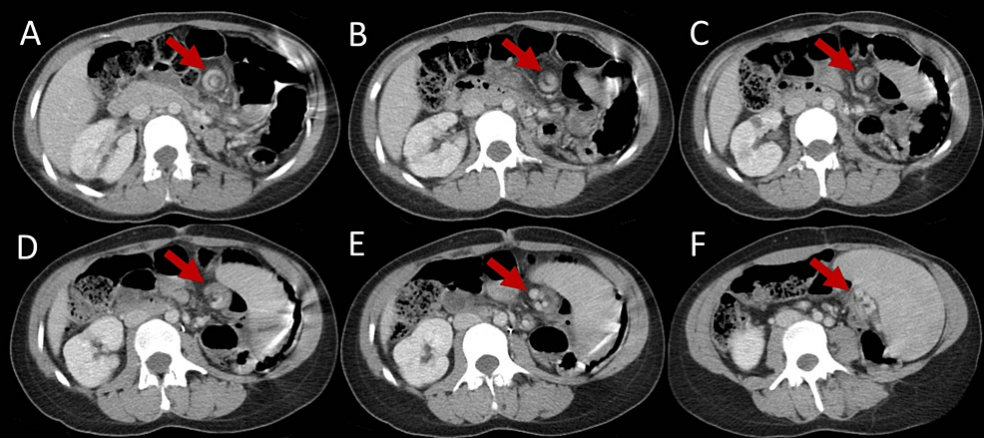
The "Whirl sign" from CT is depicted by red arrows. Images A-F show the progression of transverse CT acquisitions.
